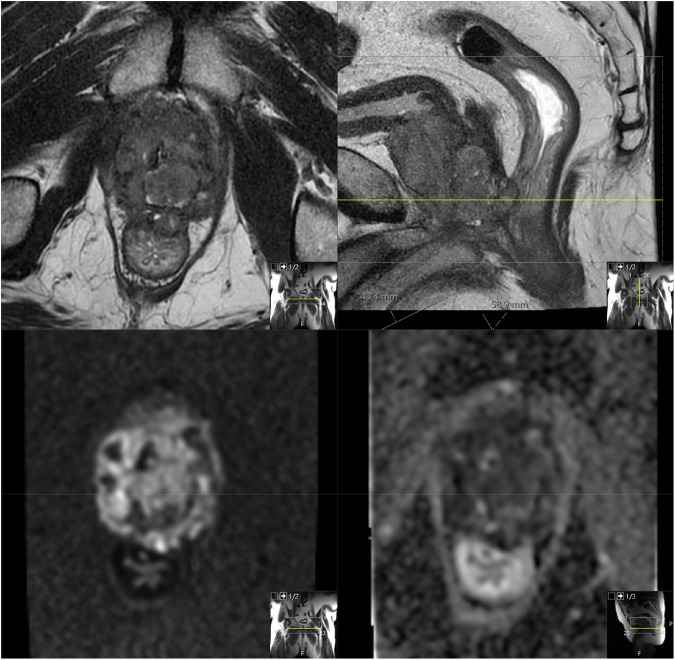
Locally advanced prostate cancer with rectal wall invasion (T4). MRI demonstrates a large infiltrative posterior prostate lesion with low T2 signal intensity and ill-defined margins, extending beyond the posterior prostatic borders into the anterior rectal wall. There is obliteration of the rectoprostatic fat plane, with direct tumour contact and infiltration of the rectal muscularis propria, associated with marked diffusion restriction. These findings are consistent with direct invasion of adjacent organs (T4 disease). Patient underwent radiotherapy and long-term hormonal therapy
